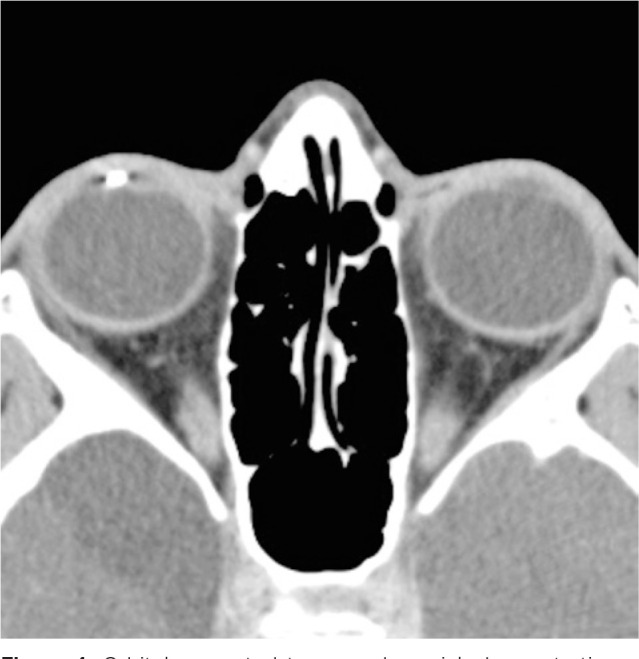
Orbital computed tomography, axial, demonstrating a metallic foreign body
